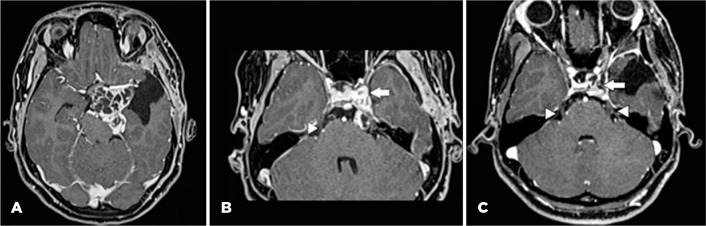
(A) Preoperative brain magnetic resonance image showing an expansive
